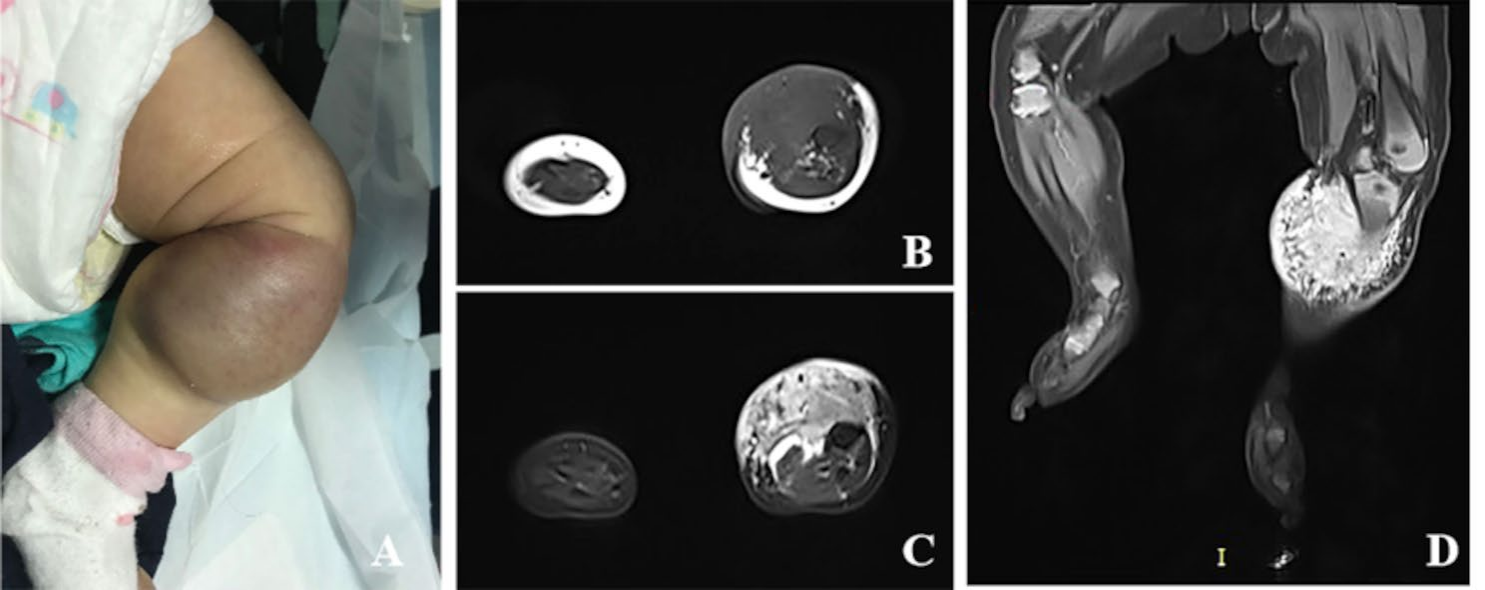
MRI images. A: Appearance of KHE in a 2-month-old female infant’s left leg; B: Coronal T1WI of the left leg shows a low-signal soft tissue mass surrounding the middle segment of the humerus; C: PDWI shows the mass to be hyperintense; D: Coronal contrast-enhanced FS T1WI reveals marked heterogeneous enhancement of the mass
